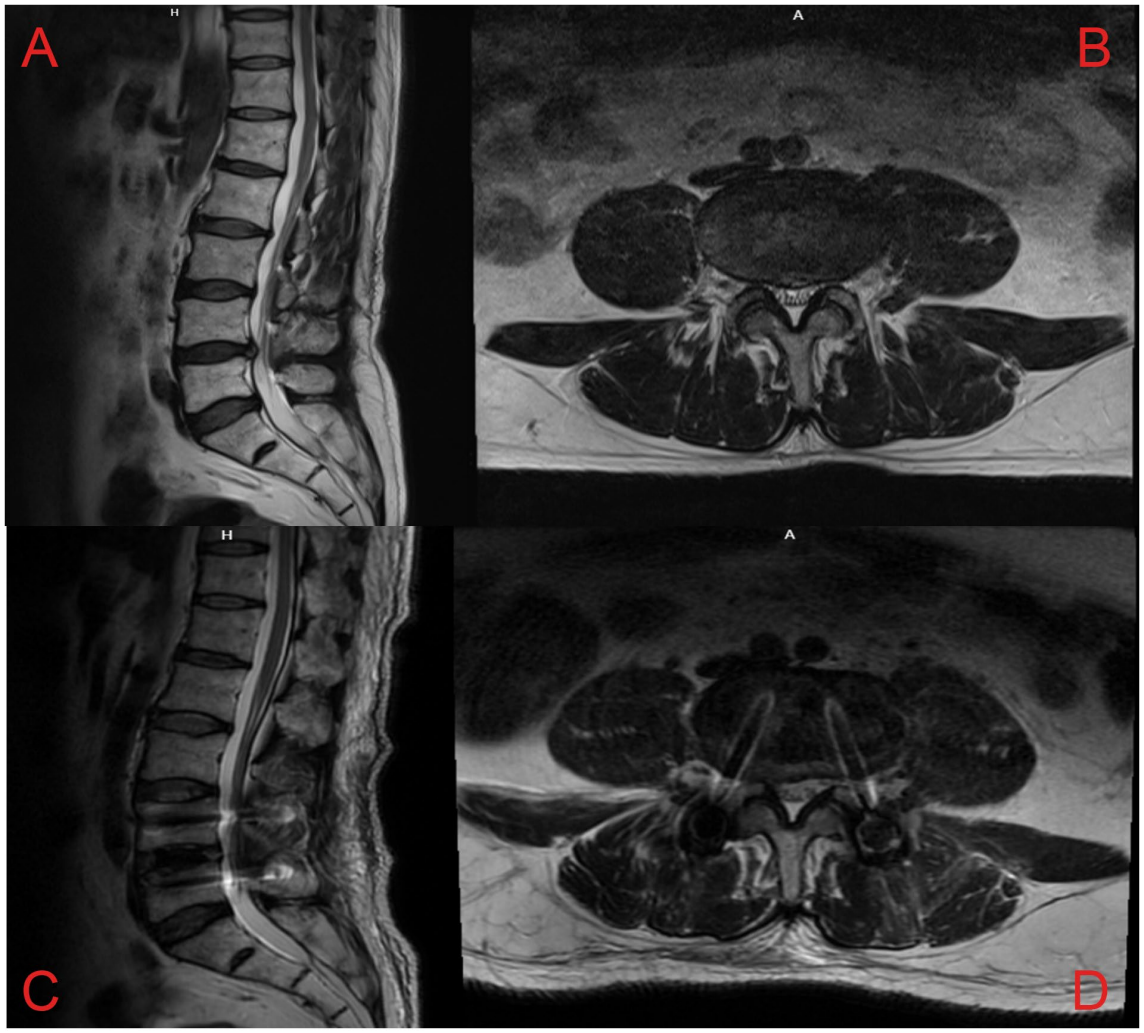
Lumbar spine MRI scans of a patient with pain and numbness in both lower extremities due to a herniated disc at L4/5. Preoperative lumbar MRI: (A) Sagittal T2W MRI revealed a herniated lumbar disc and decreased signal in the intervertebral disc at L4/5, (B) Axial T2W MRI revealed the herniated disc at L4/5. Postoperative lumbar MRI: Sagittal (C) and axial (D) images revealed the compression of the spinal cord by the intervertebral disc relieved at L4/5, and the reduction screws were fixed.
